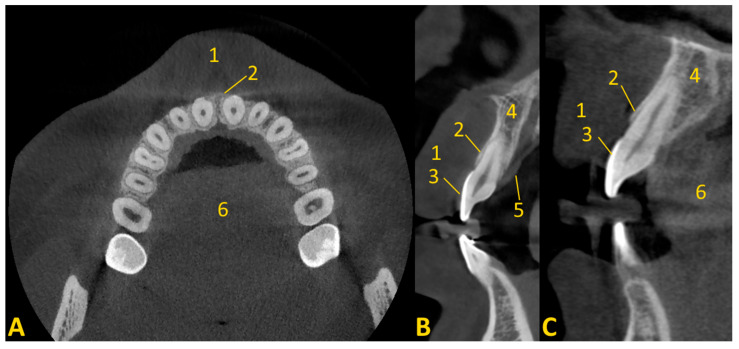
Cone beam computed tomography without soft tissue maneuver (invisible outline of the marginal gingiva from the vestibule side). Cases of soft tissue retraction, after viewing the CBCT images, can be assessed to clearly distinguish between the gingiva and the soft tissue on the palate. In cases where soft tissue retraction is not available, we are unable to clearly differentiate between the lip and gingiva: (A) axial view; (B,C) cross-sectional view. 1—lip; 2—bone edge margin; 3—enamel; 4—alveolar bone; 5—soft tissue of palate; 6—tongue.
